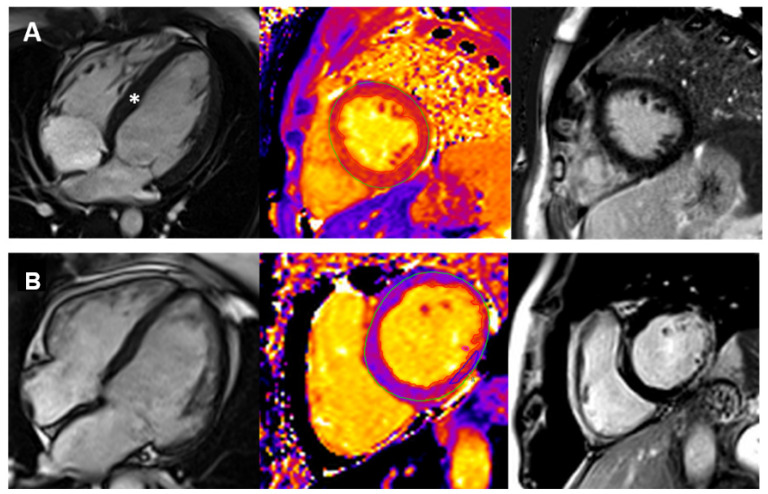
Cardiovascular Magnetic Resonance Imaging in three patients with different cardiomyopathy phenotypes. Panel (A): early hypertrophic cardiomyopathy (HCM). There is concentric left ventricular hypertrophy (left), accompanied by prolonged T1 mapping values (center) and no late gadolinium enhancement. The white asterisk (left) indicates the hypertrophic septum. Panel (B): dilated cardiomyopathy (DCM) in a patient with Duchenne muscular dystrophy. There is moderate chamber enlargement and LV systolic dysfunction (LVEF 37%) (left). T1 mapping values are globally elevated (center), and there is mainly epicardial late enhancement of the basal to mid lateral wall (right).
